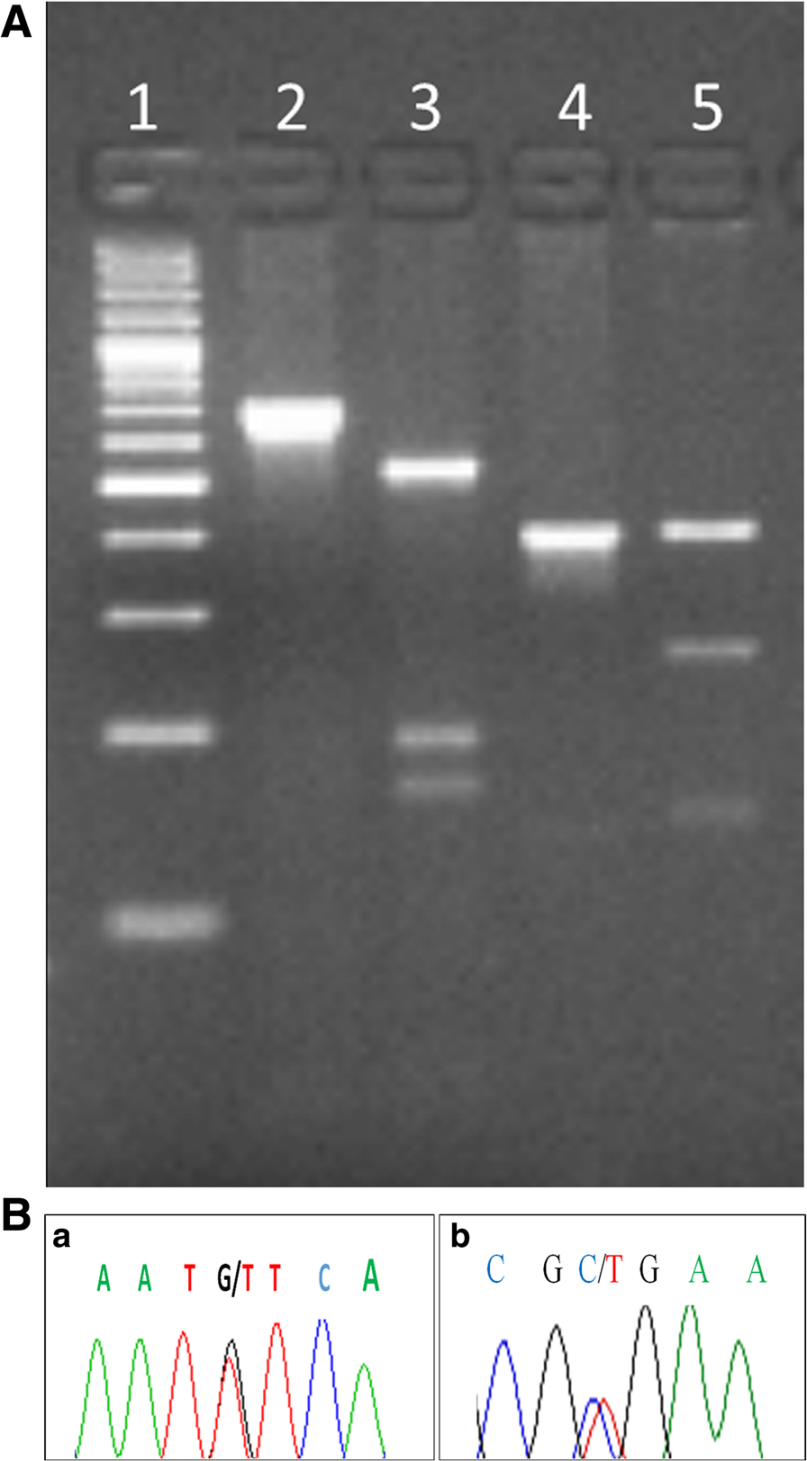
a IL-28B polymorphism showing host genotype CT (rs12978960) and TG (rs8099917) in the patient. Line 1: DNA ladder; Line 2: Amplified uncut product of rs12978960; Line 3: CT genotype by RFLP; Line 4: Amplified uncut product of rs8099917; Line 5: GT genotype by Restriction Fragment Length Polymorphism (RFLP). b Chromatogram showing (a) heterozygous G/T (rs8099917) and (b) heterozygous C/T (rs12979860) polymorphism of IL28b
